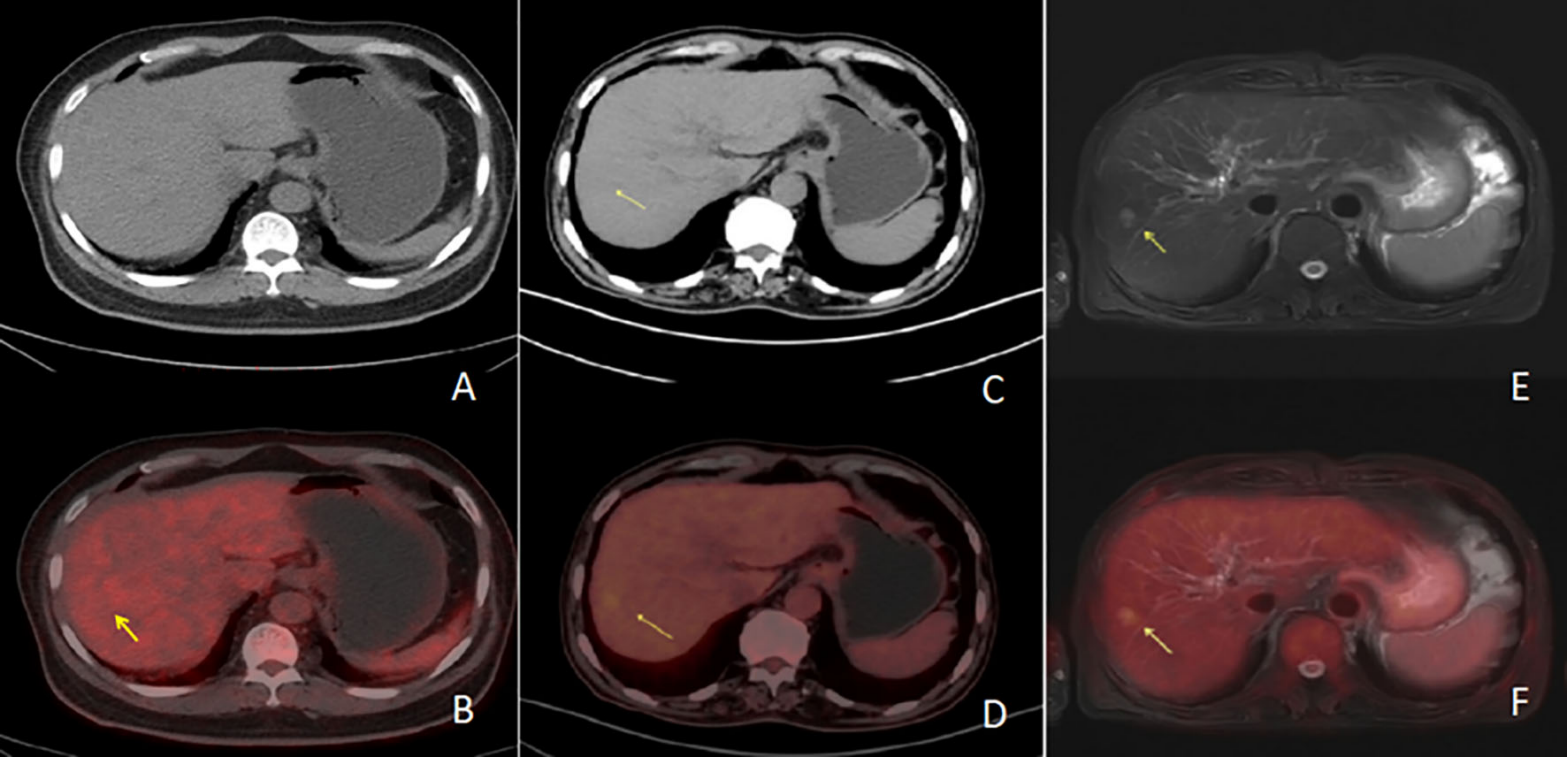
Row 1 (A, B, E) shows PET/CT images [(A) is a PET/CT CT image, (B) is a PET/CT fusion image, and (E) is a PET/CT PET image], and the second row (C, D, F) shows PET/MR images [(C) is a PET/MR MR image, (D) is a PET/MR fusion image, and (F) is a PET/MR PET image]. The CT image of the PET/CT lesions showed hypointensity with increased metabolism and a SUVmax of 6.4. In PET/MR images, T2WI lesions showed high signal with increased metabolism and a SUVmax of 3.7.
